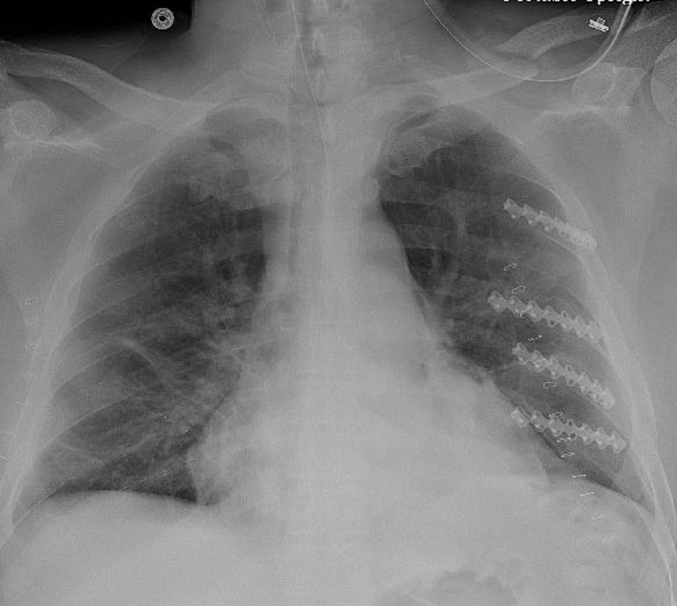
Postoperative chest X-ray demonstrating final hardware and bilateral lung expansion after chest tube removal.
